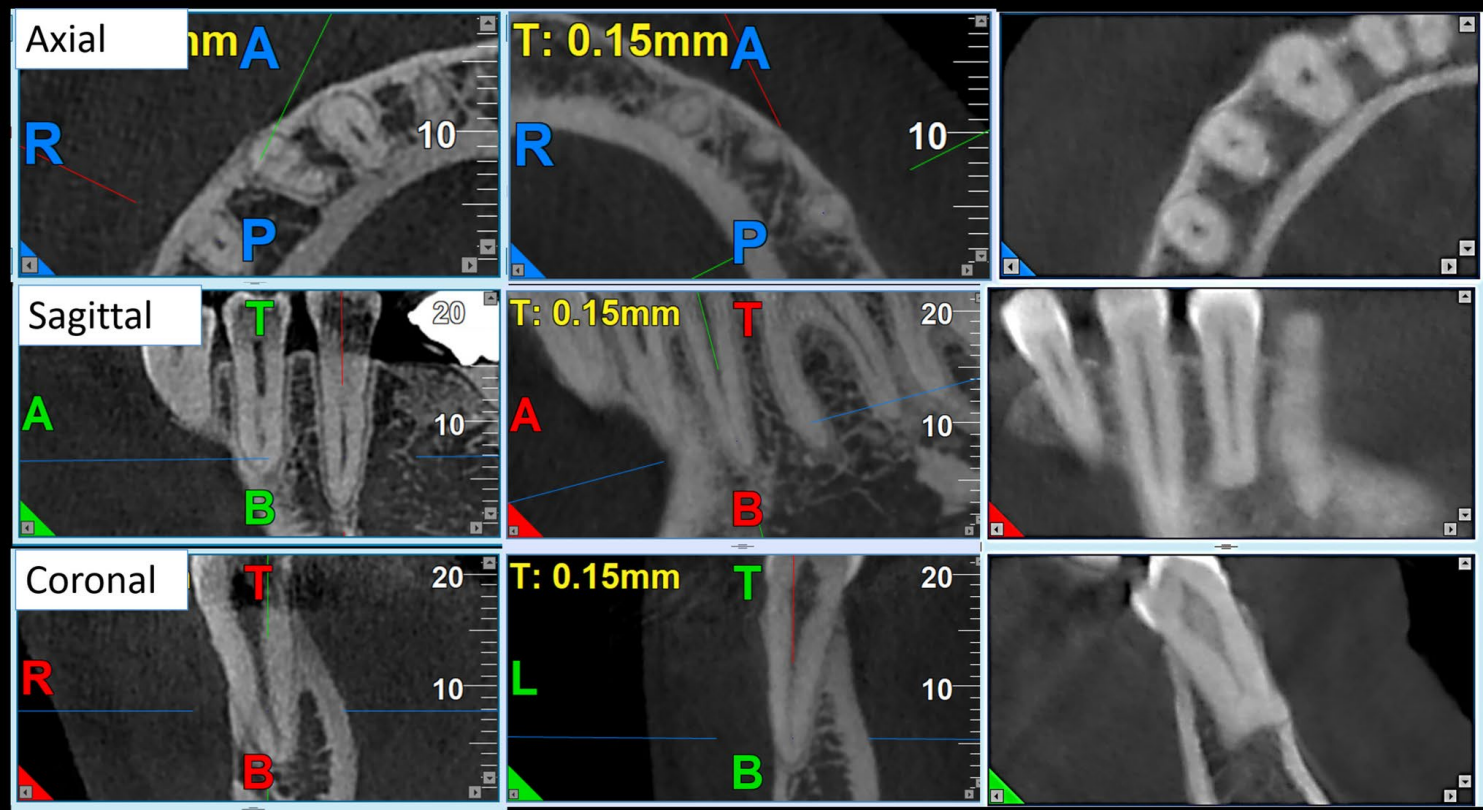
CBCT images of single-rooted mandibular second premolars in axial, sagittal, and coronal views
